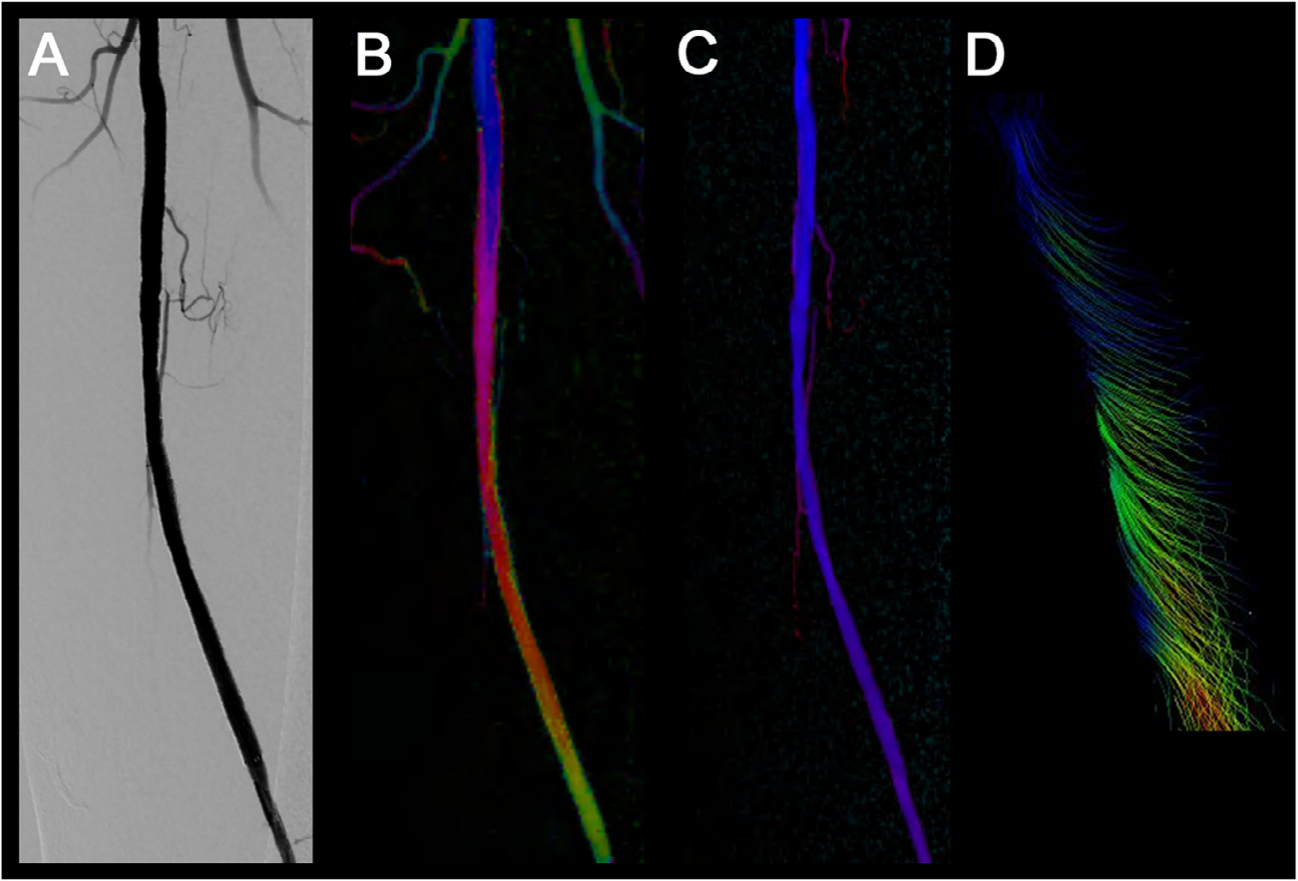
Color-coded circulation image before and after stent placement. (A) Digital subtraction angiography (DSA) image after stent placement. (B) Color-coded circulation image after stent placement. (C) Color-coded circulation image before stent placement. (D) Streamline image by 4-dimensional flow magnetic resonance imaging at the stent exit site. It was difficult to confirm the presence of swirling flow using DSA (A). Layered colors are observed at the stent site (B, supplementary material: video displays) but are not present before stent placement (C). Swirling flow is visible in the streamlined image (D).
